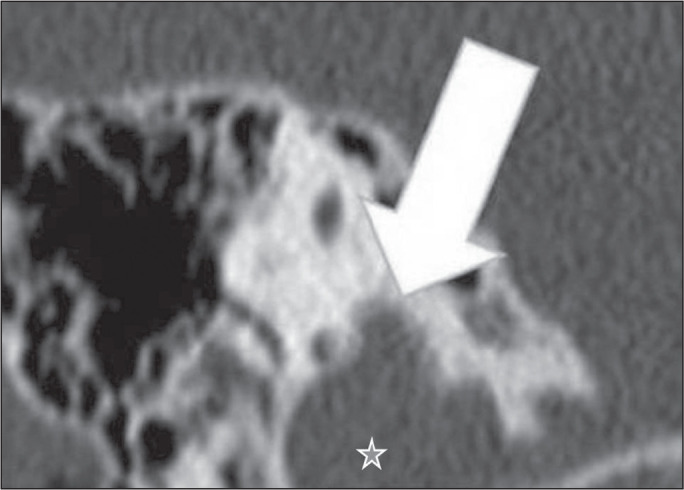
Jugular bulb diverticulum in 38-year-old woman. Unenhanced coronal CT
